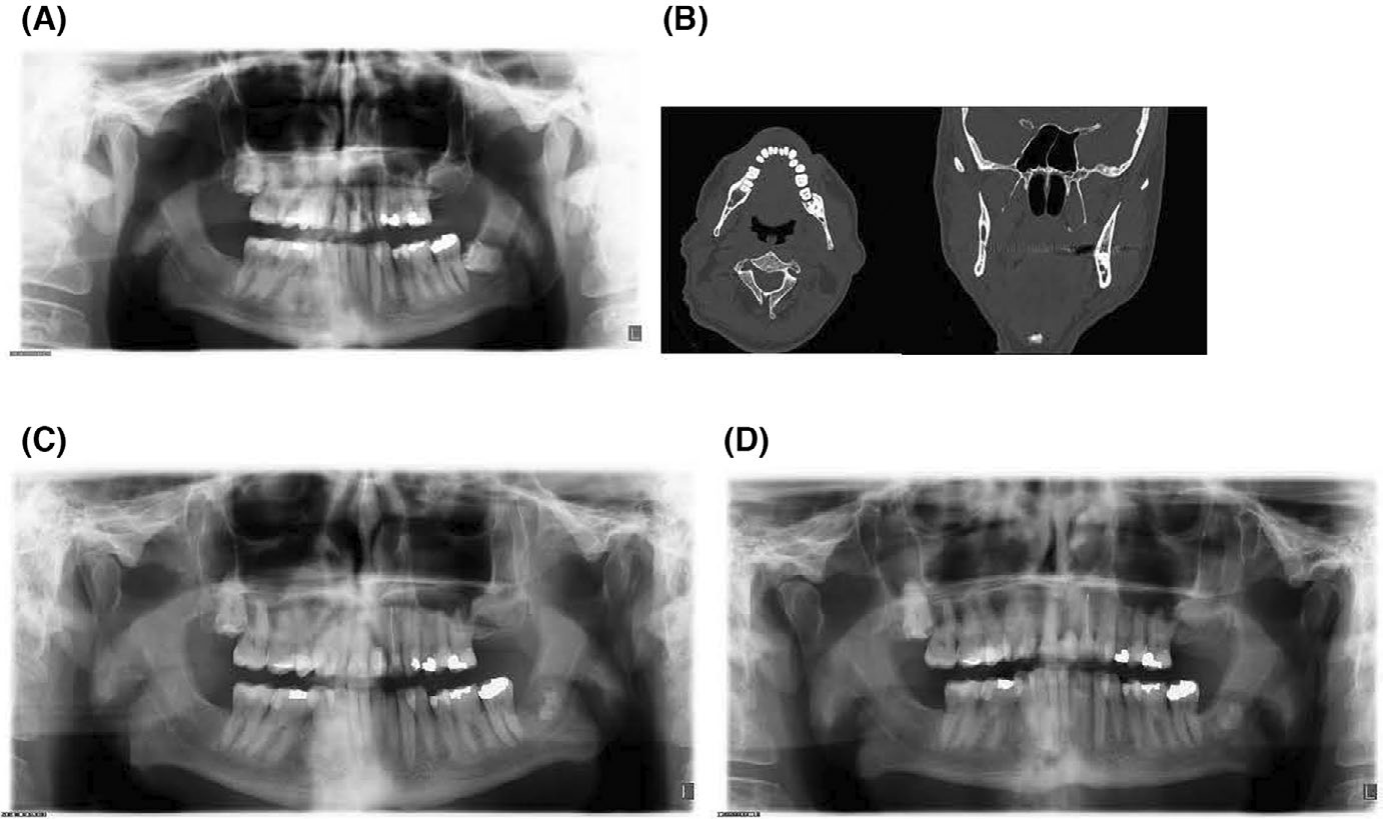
The preoperative X‐orthopantomogram (X‐OPT) (A), a preoperative CT scan (B), a postoperative X‐OPT at 6 months after coronectomy (C) and a postoperative X‐OPT at 1 year after coronectomy (D) in one of the included patients.
